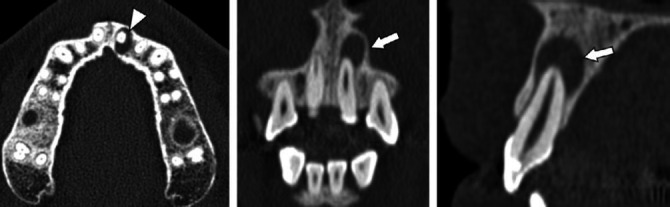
Radicular cyst in a 37-year-old female patient. CT images showing a
